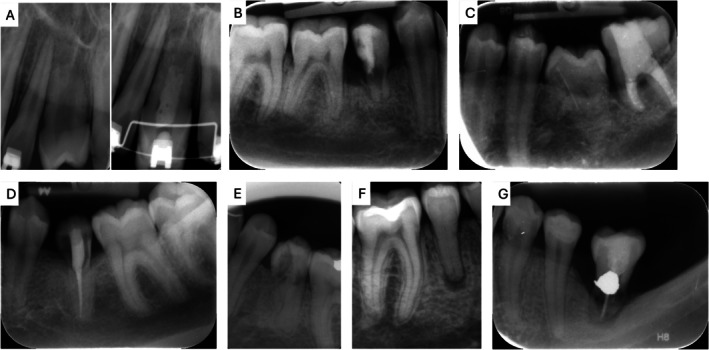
Representative radiographic examples of the main post‐operative complications. (A) External inflammatory resorption (EIR): initial diagnosis (left), first application of calcium hydroxide (right); (B) Internal inflammatory resorption (IIR); (C) Replacement resorption (RR); (D) External cervical resorption (ECR) with vascular soft‐tissue ingrowth; (E) ECR with osseous tissue ingrowth; (F) Apical pathology (AP); (G) Periodontal attachment loss (AL) with concurrent resorption.
